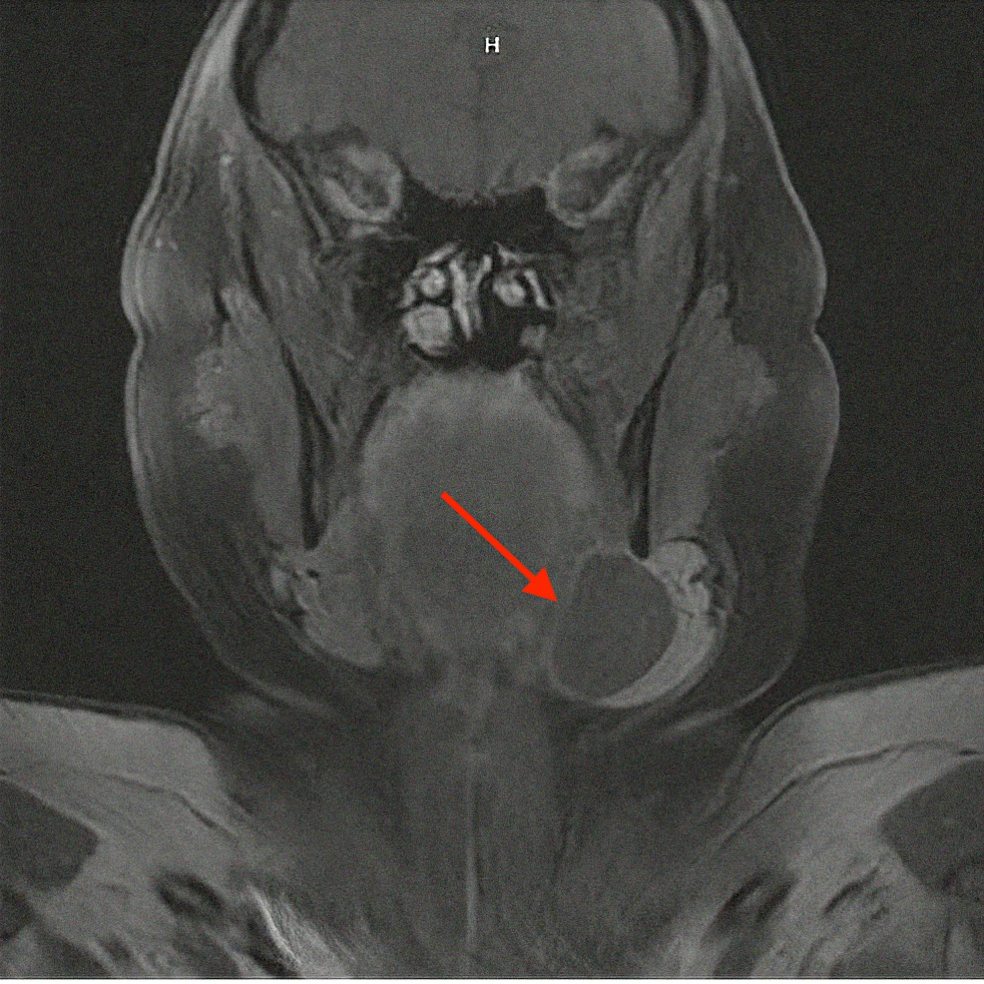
T1-weighted MRI of the head and neck with contrast, coronal cut, demonstrating a thin-walled, nonenhancing, elongated cystic mass (red arrow) measuring 4.8 cm x 2.6 cm on the medial aspect of the left submandibular gland.
